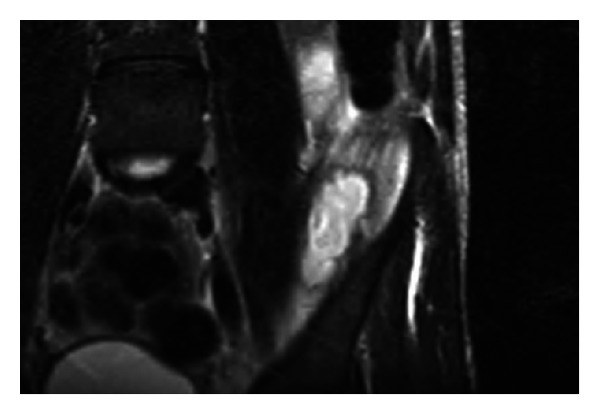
MRI image of iliacus abscess.
